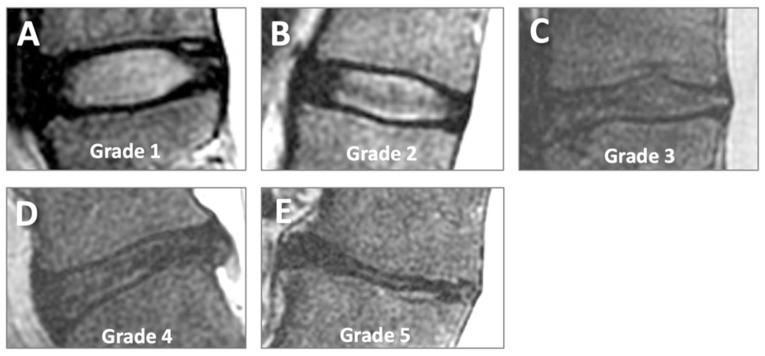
Pfirrmann grading of disc degeneration on sagittal conventional T2-weighted MR images. Grades 1 (A), 2 (B), 3 (C), 4 (D), and 5 (E) represent increasing severity of disc degeneration.
